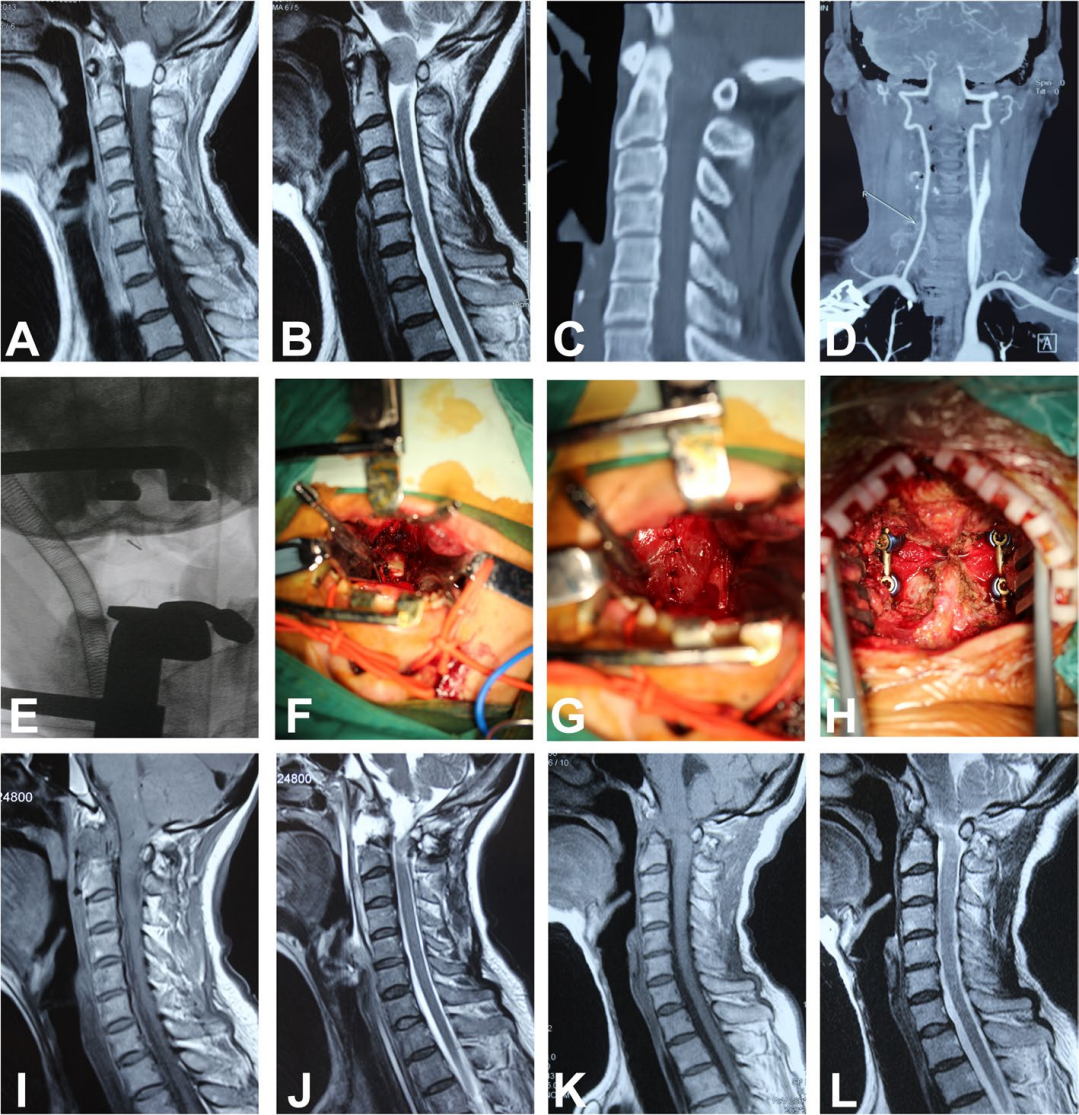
A 55-year-old woman presented with numbness and weakness of limbs and was diagnosis with basilar invagination. A and B: Preoperative sagittal T1-weighted and T2-weighted MR images show intraspinal tumor in the upper cervical spine. C and D: Preoperative sagittal CT scan and CT angiography of the upper cervical spine. E: Intraoperative fluoroscopy with locating pin. F: Intraoperative tumor exposure after spinal durotomy. G: Duraplasty after tumor excision. H: Posterior fusion and stabilization. I and J: Postoperative sagittal T1-weighted and T2-weighted MR images show that intraspinal tumor was resected and the spinal cord was sufficient decompressed. K and L: Sagittal T1-weighted and T2-weighted MR images at a follow-up of 3 months postoperatively and the patient could walk well after the operation
